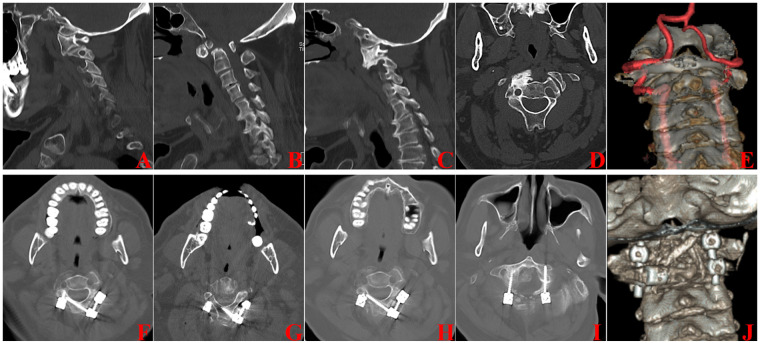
A 48-year-old male: (A–E) CT and vertebral arteriography showed atlantoaxial dislocation, free odontoid process and high span vertebral artery; (F–J) CT performed 2 years after surgery revealed well-positioned C2 laminar screws with intact screw-to-screw connections.
